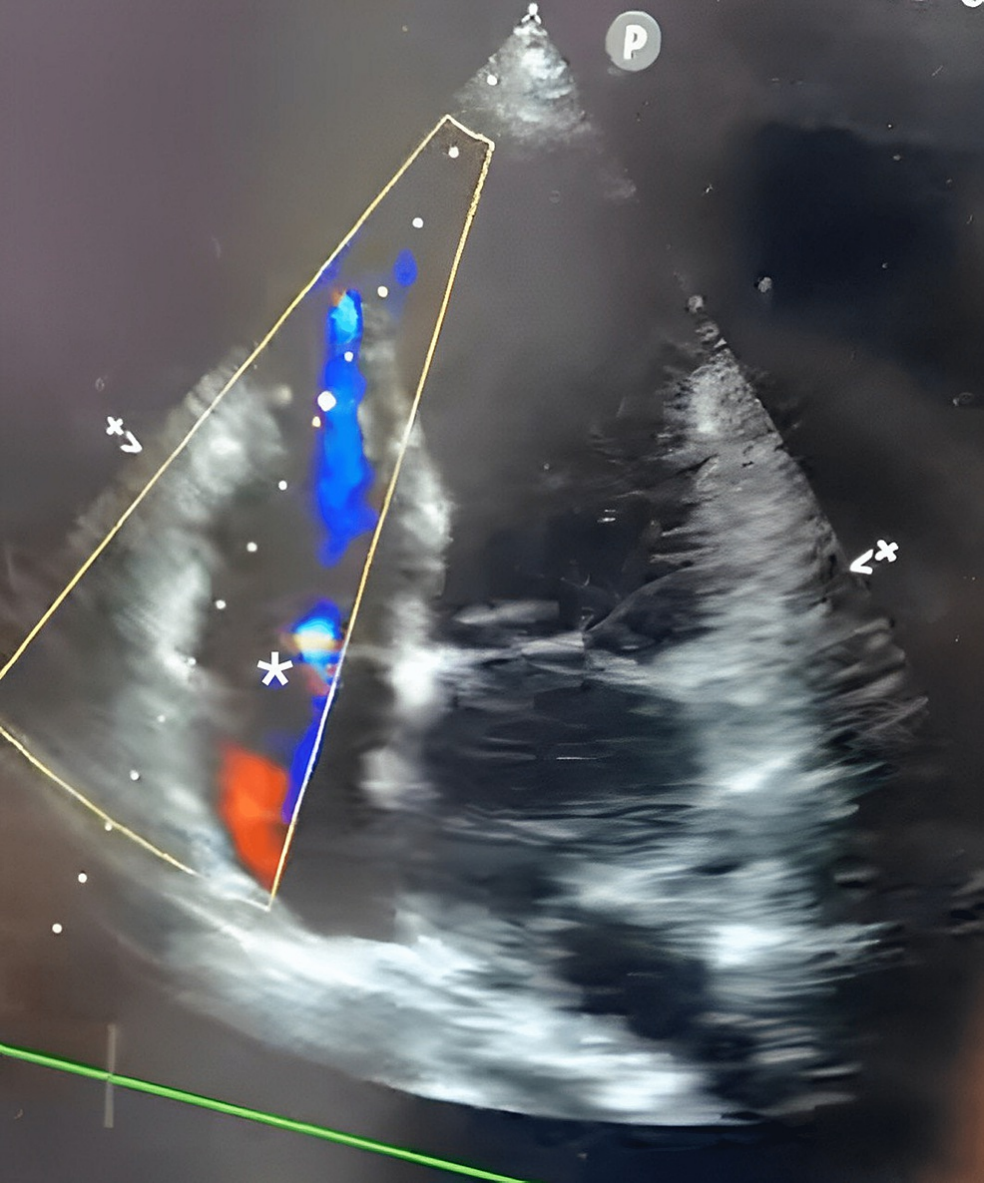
A presence of flow is seen on color Doppler across the interventricular septum from the left to the right ventricle * marks the region of interest
